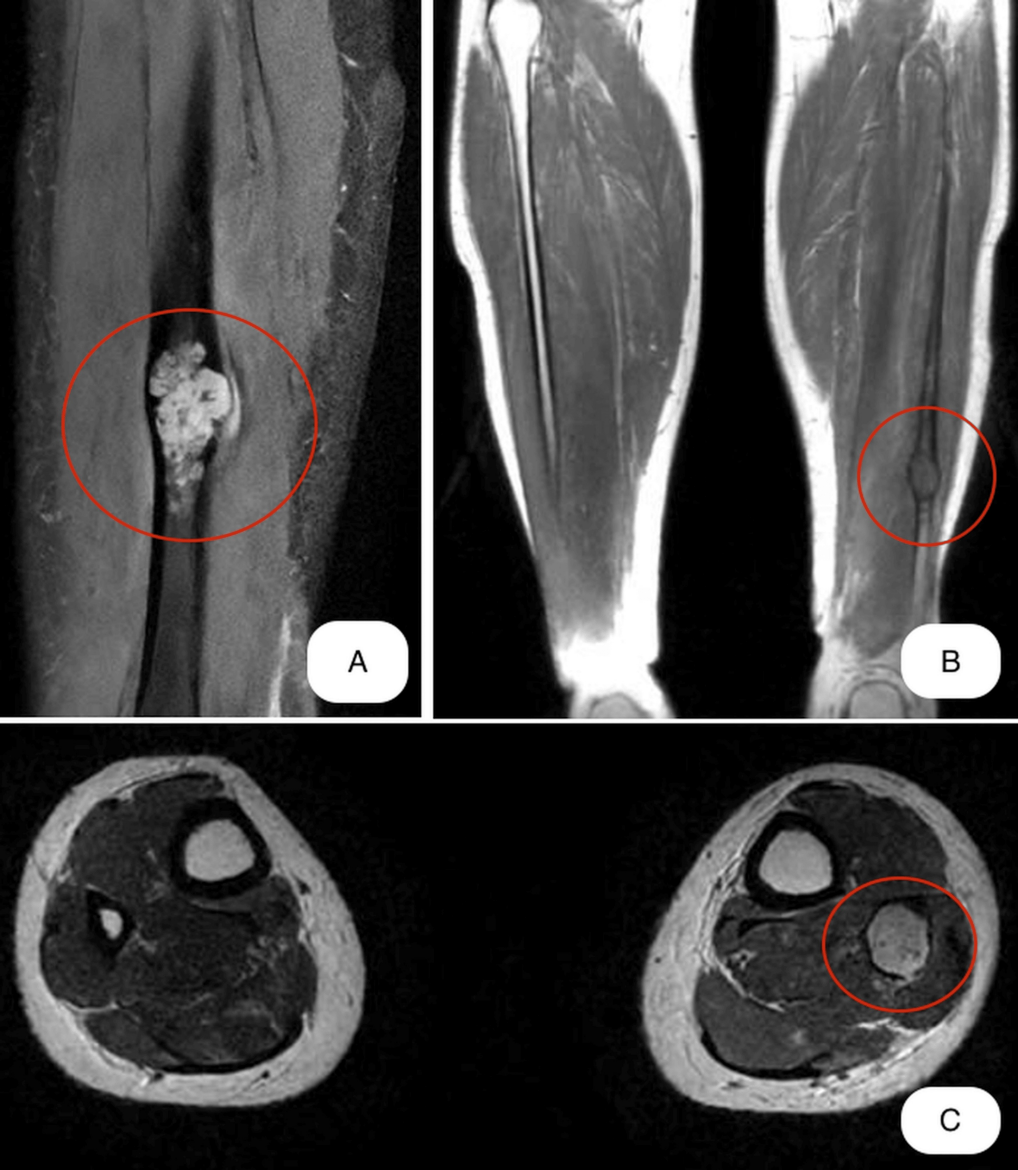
Magnetic resonance imaging (MRI) distal 1/3 of the fibula shaft before surgery A - sagittal view B - coronal view C - axial view
